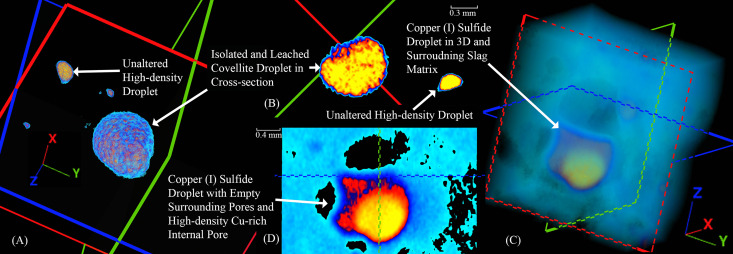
Two-dimensional and 3D images of slags -S37A [(A), (B)] and H76-S45B [(C), (D)], respectively, showing internal metal droplets. (A, B) Cross-section of the large covellite droplet showing variably dense but distinguishable phases. In contrast, note that the smaller, unaltered droplet immediately next to it, which has no connecting conduits, is contiguous and shows no signs of leaching or corrosion. (C, D) CT scan aligning with the sectioned droplet. Note the three external pores and the high-density internal pore of the droplet. The internal pore has a surface layer of cuprite, which attenuates the X-rays from the CT scan, giving it a more intense red color.
